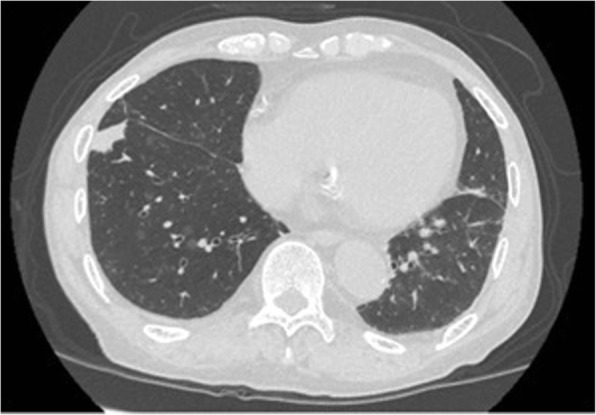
Chest CT scan before TAVI
